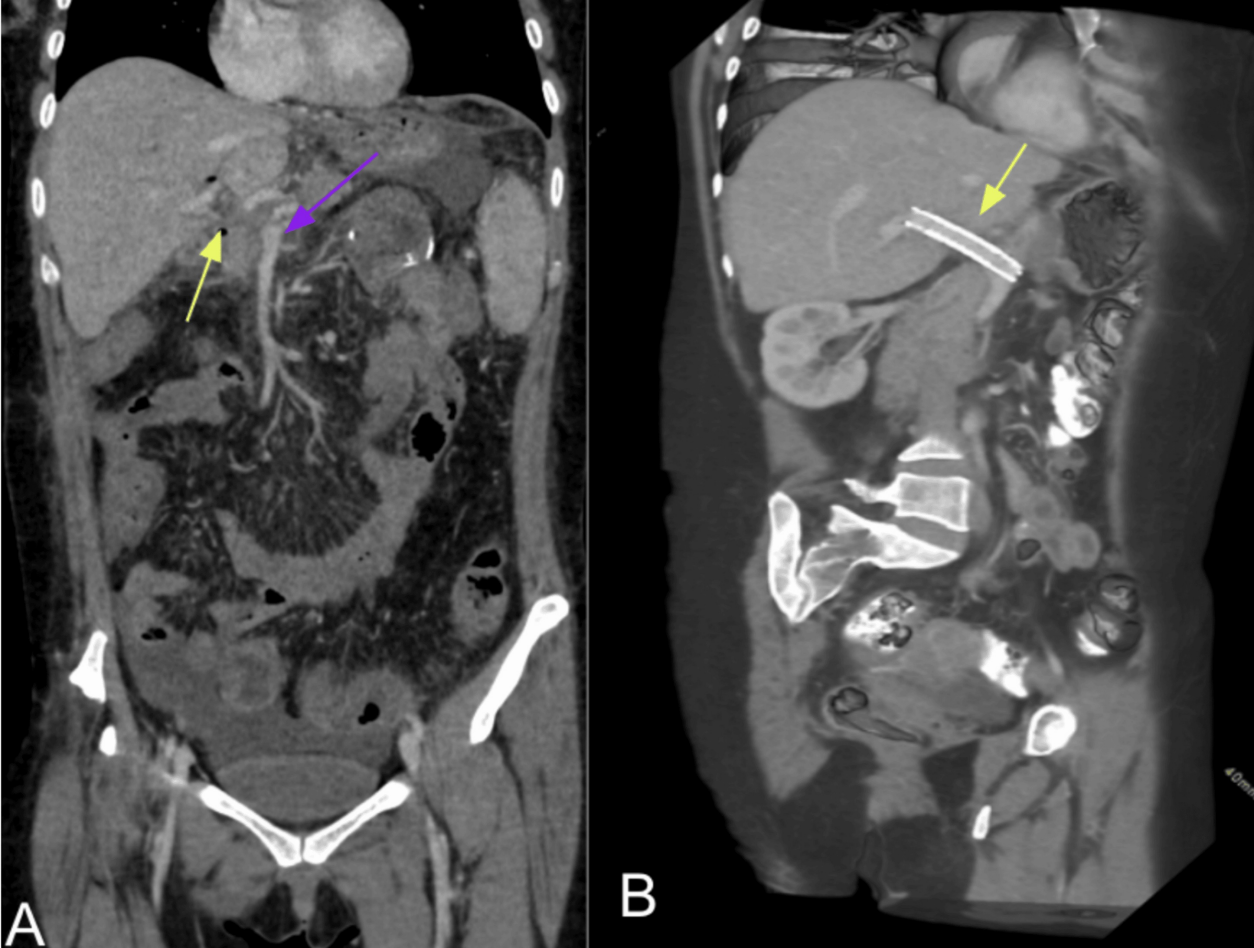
Contrast-enhanced abdominal CT angiography (A) Coronal reconstruction shows diffuse colitis involving the ascending, transverse, descending, and sigmoid colon, associated with pericolic fat stranding. The liver graft demonstrates atrophy of the left hepatic lobe (purple arrow) with compensatory hypertrophy of the right lobe. Cavernous transformation of the portal vein is identified (yellow arrow), secondary to chronic thrombosis, with no evidence of main portal vein recanalization. Additional signs of portal hypertension include perisplenic collateral vessels, splenomegaly, perigastric and mesenteric varices, and ascites in the perihepatic, perisplenic, paracolic, and pelvic spaces. (B) Sagittal reconstruction depicts a metallic stent at the hepatic hilum (yellow arrow), corresponding to a previously placed transjugular intrahepatic portosystemic shunt (TIPS), as part of the therapeutic strategy for portal vein thrombosis
